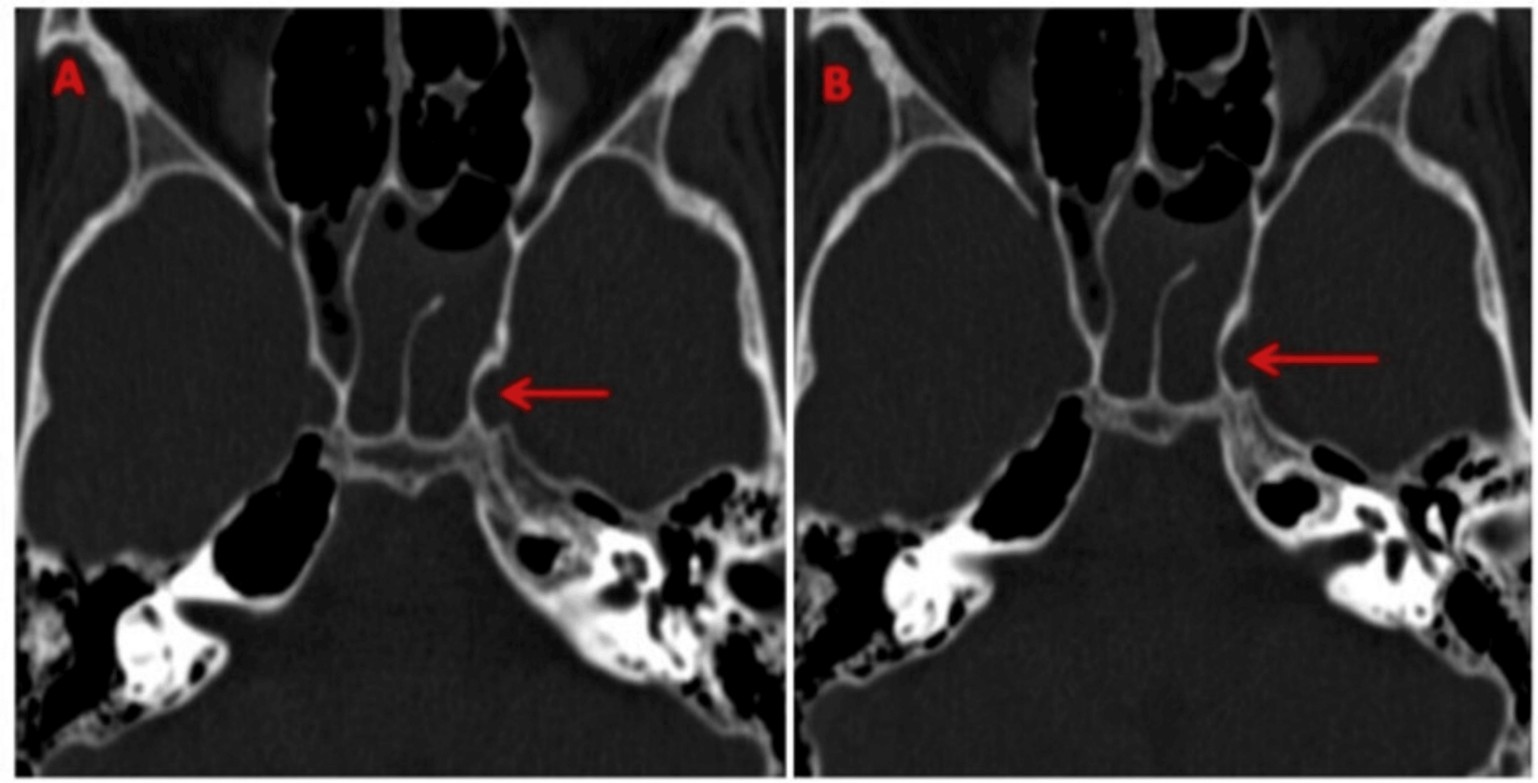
(A, B) HRCT base of skull showing proximity of the left carotid canal to the sphenoid sinus with protrusion of the carotid canal into the adjoining left sphenoid sinus (marked by red arrow). HRCT: high-resolution computed tomography.
